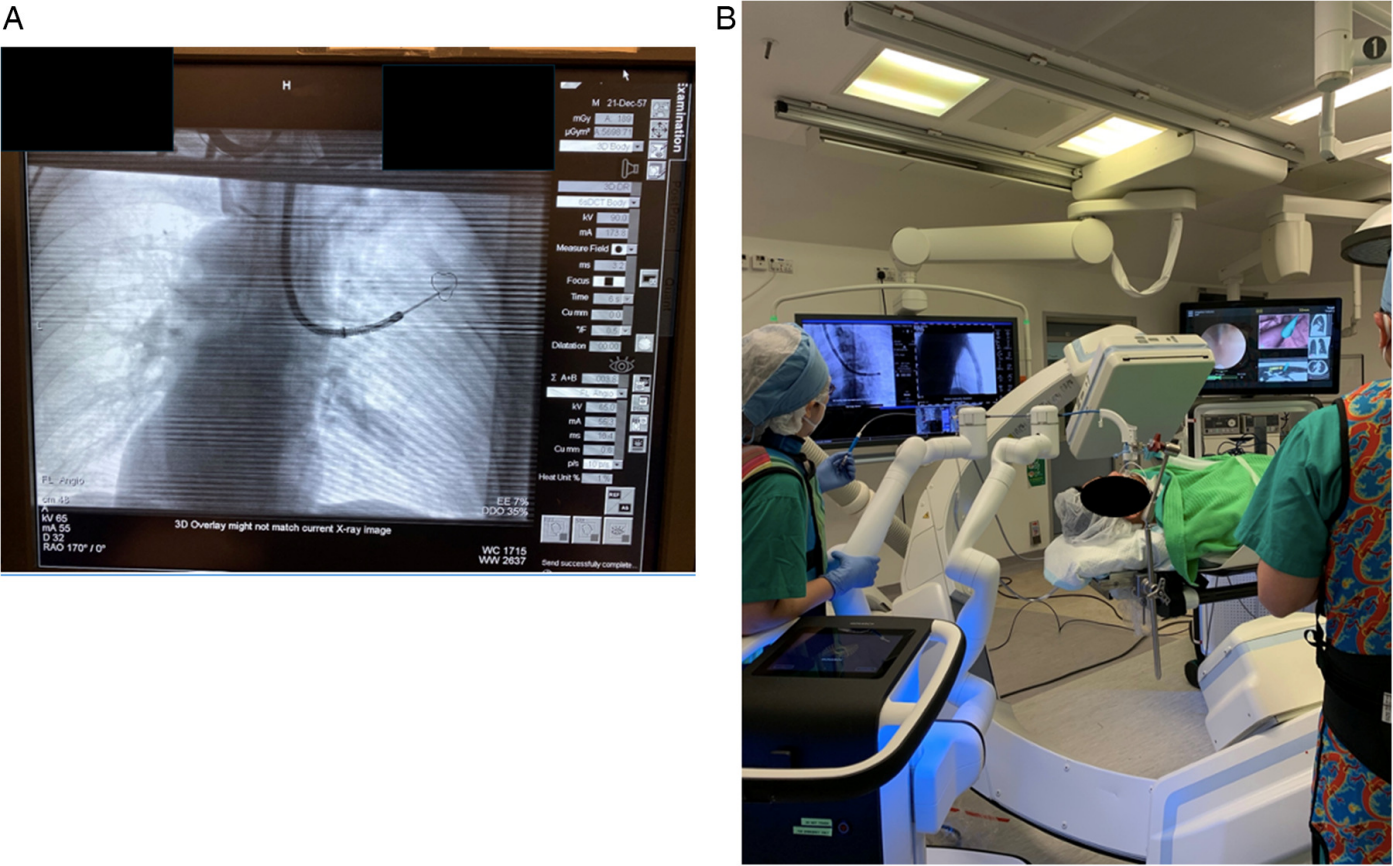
(A) Combining multimodal imaging in the HOR increases navigation accuracy and confirms the tool-in-lesion position. Real-time fluoroscopy was used to confirm the tool position during the RAB navigation. (B) Subsequently, the patient was kept in the same position, and CBCT spin was performed to confirm the tool-in-lesion position.
